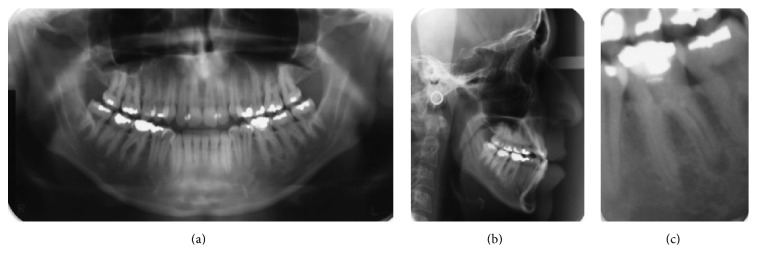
(a) Panoramic X-ray showed integrity of the permanent teeth and absence of upper third molars; (b) cephalometric analysis showed a severe vertical pattern, convex profile, increased lower facial height, a slightly retruded maxilla in relation to the cranial base, and a well-positioned mandible. The upper incisors were in a good inclination while the lower incisors were uprighted; (c) endodontic lesion in tooth 36.
